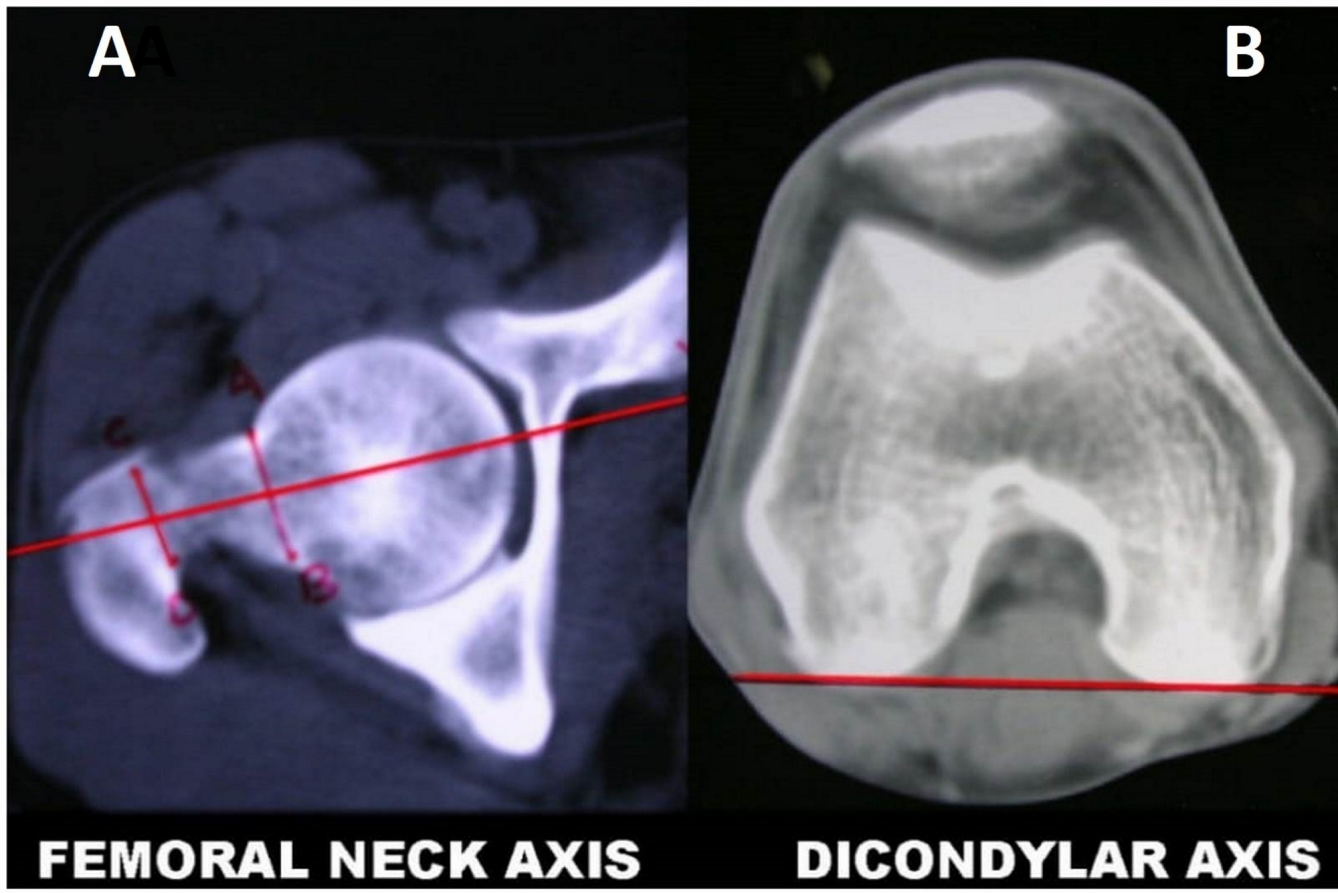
Computed tomographic evaluation A: Femoral neck axis B: Dicondylar axis
